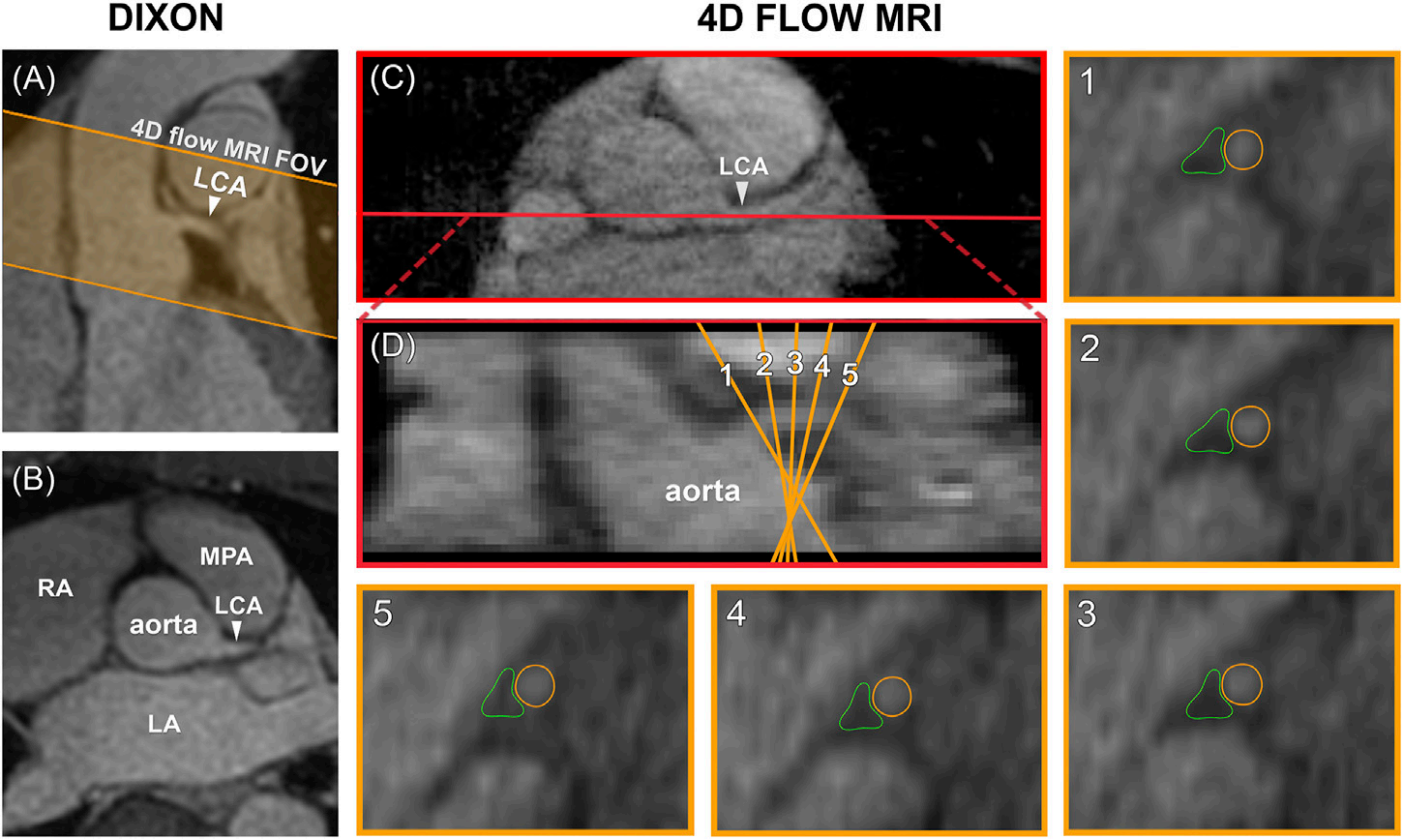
(A) Planning of the 4D flow MRI field of view (orange) on the Dixon water image. (B) Transversal view of the Dixon water image. (C) The LCA is identified on a transversal 4D flow MRI magnitude image and a longitudinal cross-section is made (shown in red). (D) The resulting coronal view is used to place five analysis planes perpendicular to the LCA. 1–5) In these planes, measurement contours are placed around the LCA lumen and in adjacent pericardial fat. FOV = field of view, LCA = left coronary artery, LA = left atrium, RA = right atrium, MPA = main pulmonary artery.
